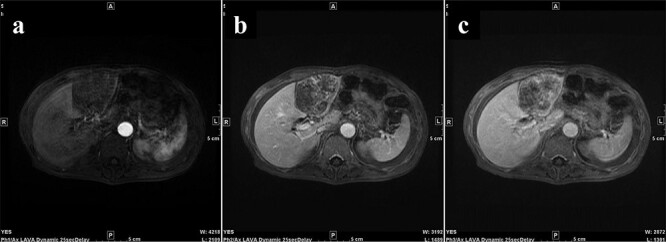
During Gd-EOB-DTPA-enhanced MRI at the time of initial hospitalization, the contrast effect of the tumor gradually increased from the peripheral part toward the hepatocyte phase from the arterial phase. (a) Arterial phase, (b) portal phase, (c) hepatocyte phase. Gd-EOB-DTPA, gadolinium ethoxybenzyl diethylenetriamine pentaacetic acid; MRI, magnetic resonance imaging.
